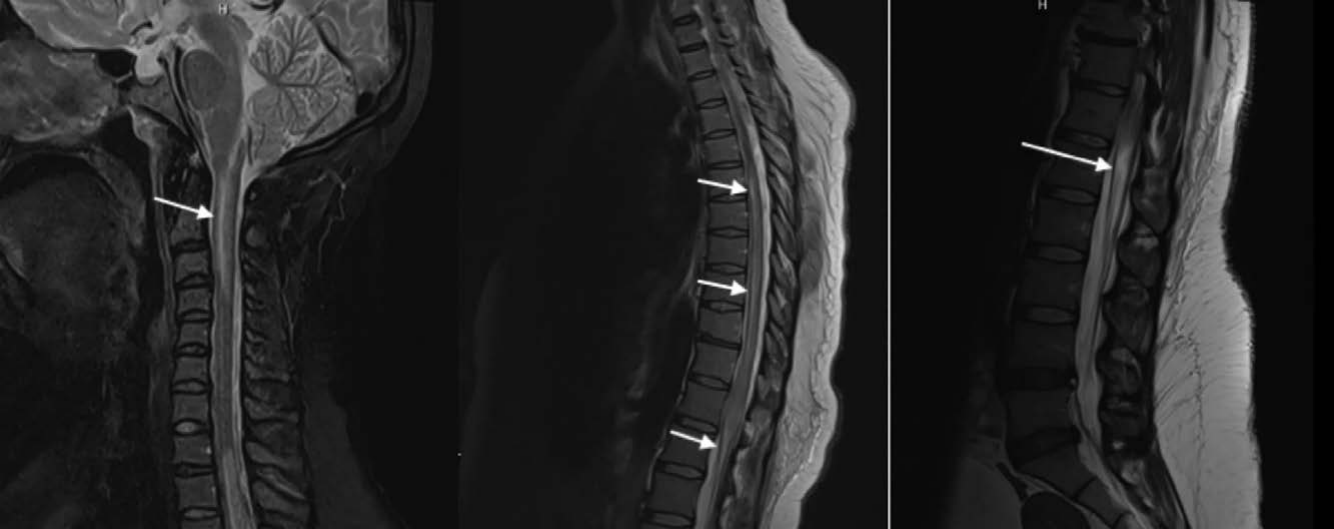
T2 MRI hyperintensity extending from the right cervical medullary junction to C4-5 interspace (image 1), and patchy enhancement extending from T5-6 to conus medullaris (images 2 and 3). MRI = magnetic resonance imaging.
